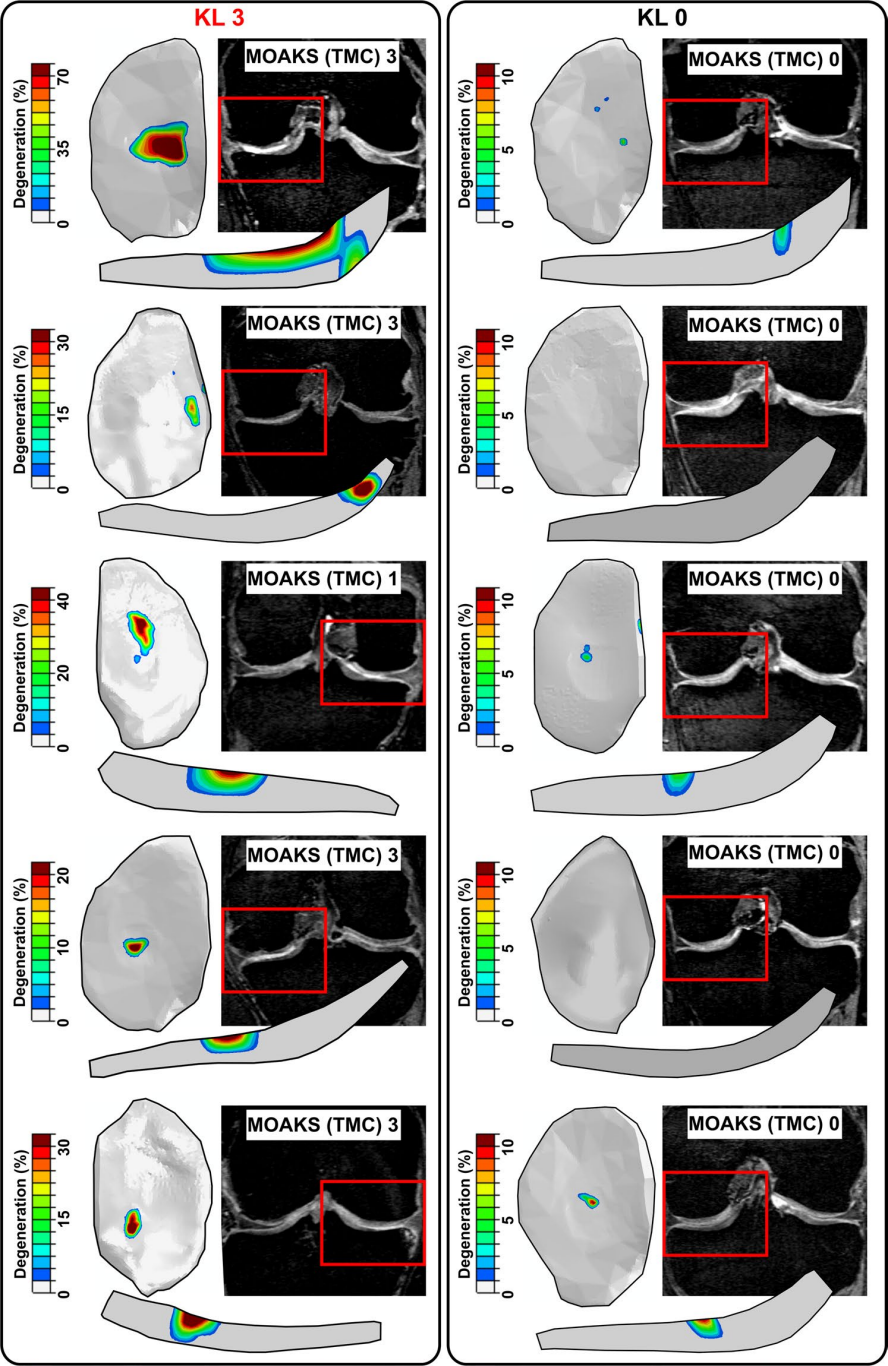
Cartilage degeneration distributions and MRI MOAKS grades for five selected KL3 and KL0 subjects. MOAKS grades are from central part of medial tibial cartilage (TMC = tibia medial central).
